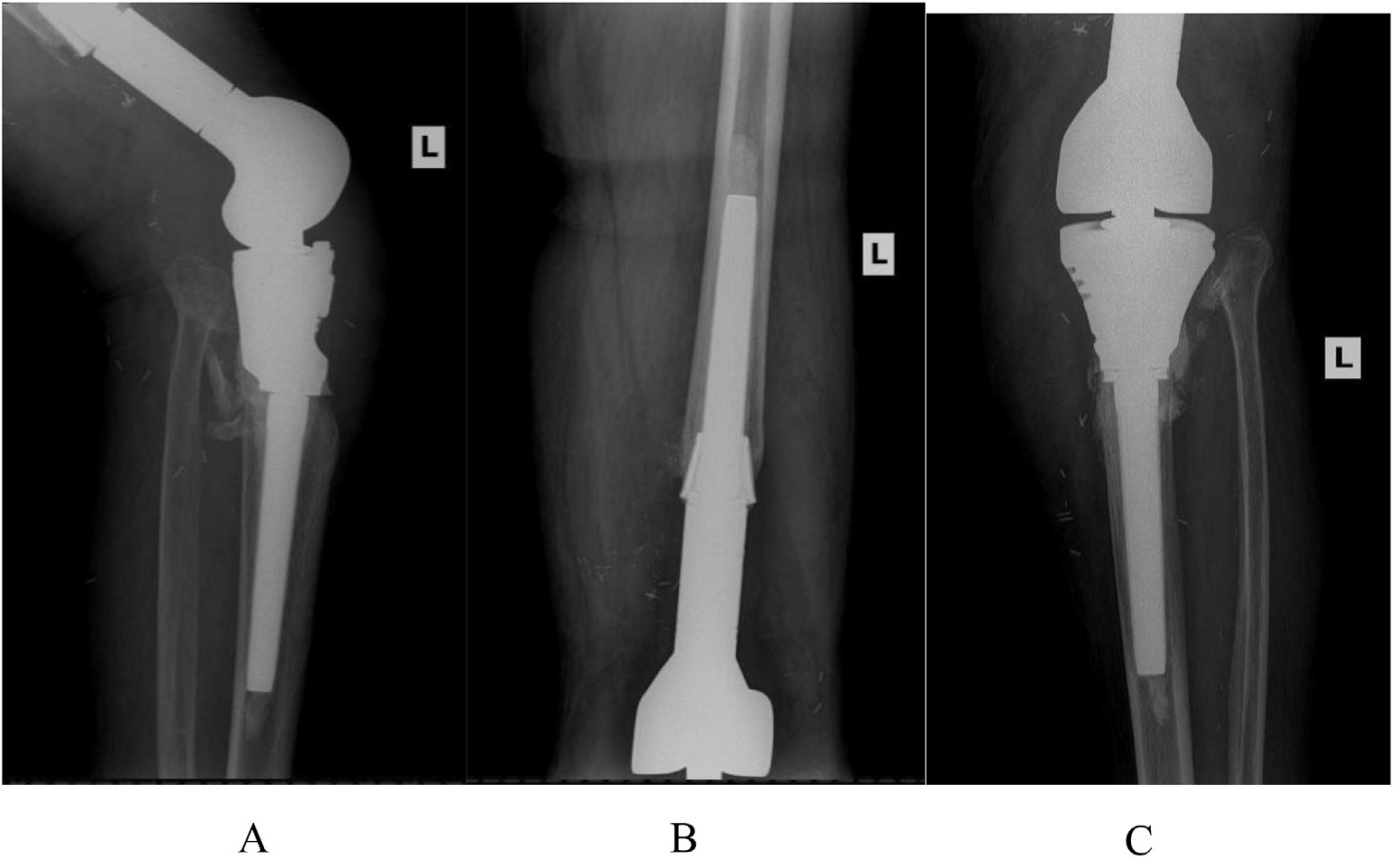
X-rays were taken prior to staging showed a well-fixed tibia and femoral stem. A. AP projection. B. Lateral projection. C. Proximal femur.
